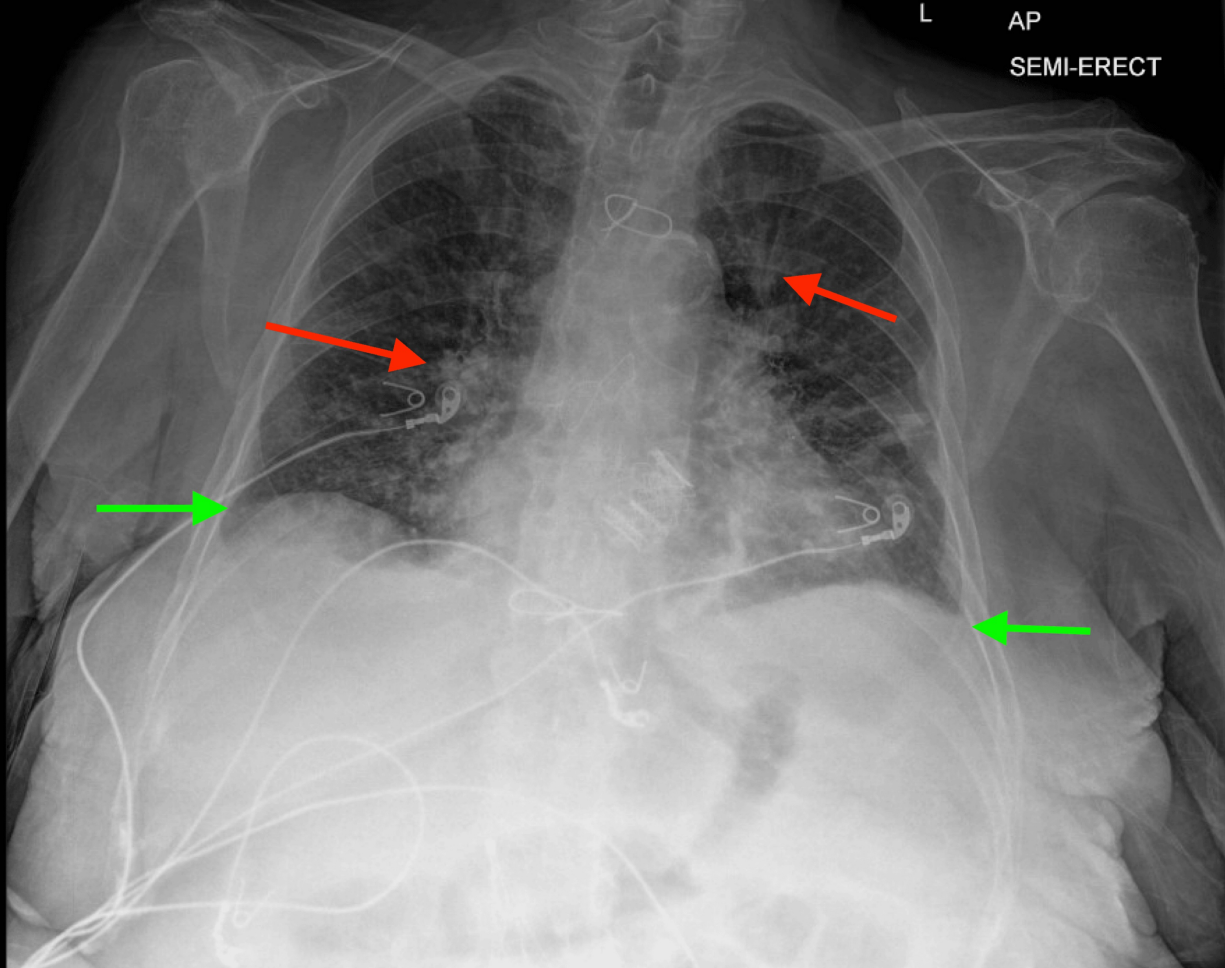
Initial chest X-ray imaging red arrows: interstitial prominence; green arrows: small pleural effusions
